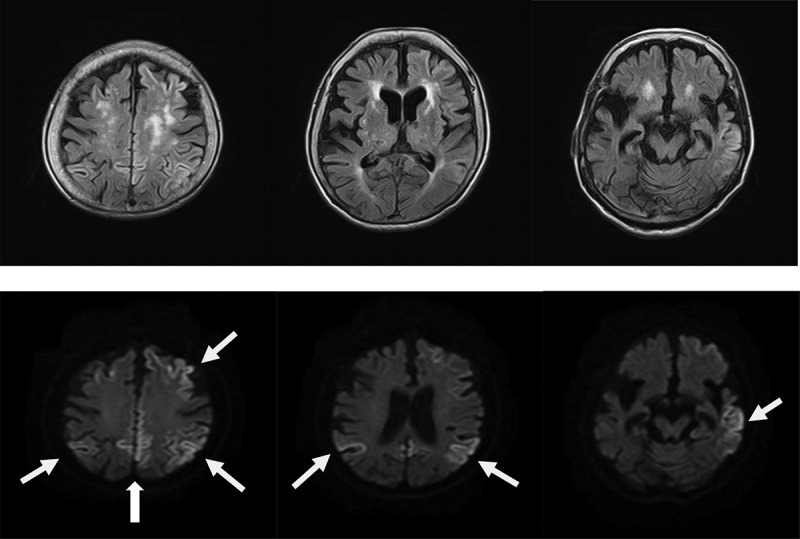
b. Magnetic resonance imaging (MRI) findings obtained using fluid-attenuated inversion-recovery (FLAIR) (upper) and diffusion-weighted images (DWI) (lower) in an 89-year-old woman at 4 months after the initial examination. DWI shows the expansion of the cortical hyperintensity areas in both hemispheres, relative to the previous findings.
